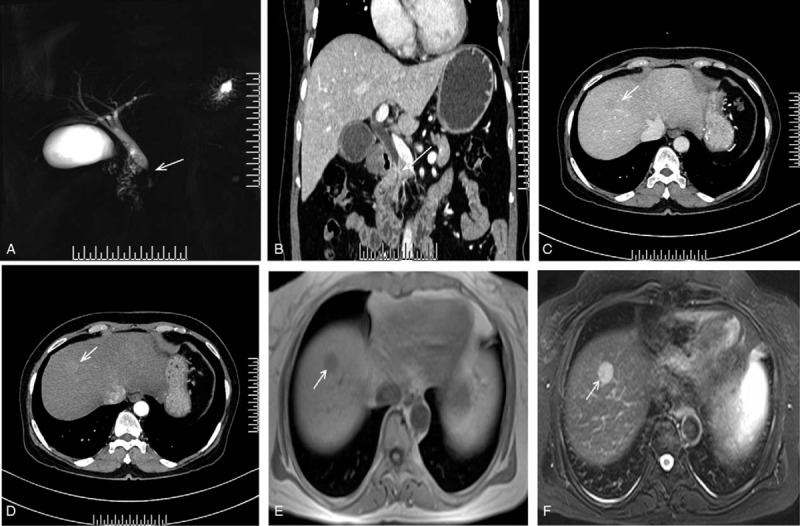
A, A MRI scan of the upper abdomen revealed the bile duct lumen is narrow (white arrow). B, A CT revealed a thickening of the bile duct wall in the middle and lower segments of common bile duct (white arrow). C, A CT scan of liver showing a low-density tumors with ring enhancement in portal phase (white arrow). D, A CT scan of liver showing a low-density tumor with ring enhancement in arterial phase (white arrow). E, Long T1 and long T2 signal nodular shadows in the parietal hepatic region with clear boundaries (white arrow). F, Long T1 and long T2 signal nodular shadows in the parietal hepatic region with clear boundaries (white arrow). CT = contrast-enhanced computed tomography, MRI = magnetic resonance imaging.
